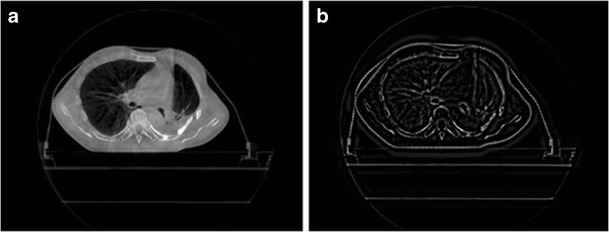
The CBCT image and the gradient image produced after the SWT. Part a is the original CBCT image and Part b is the gradient image after the SWT
